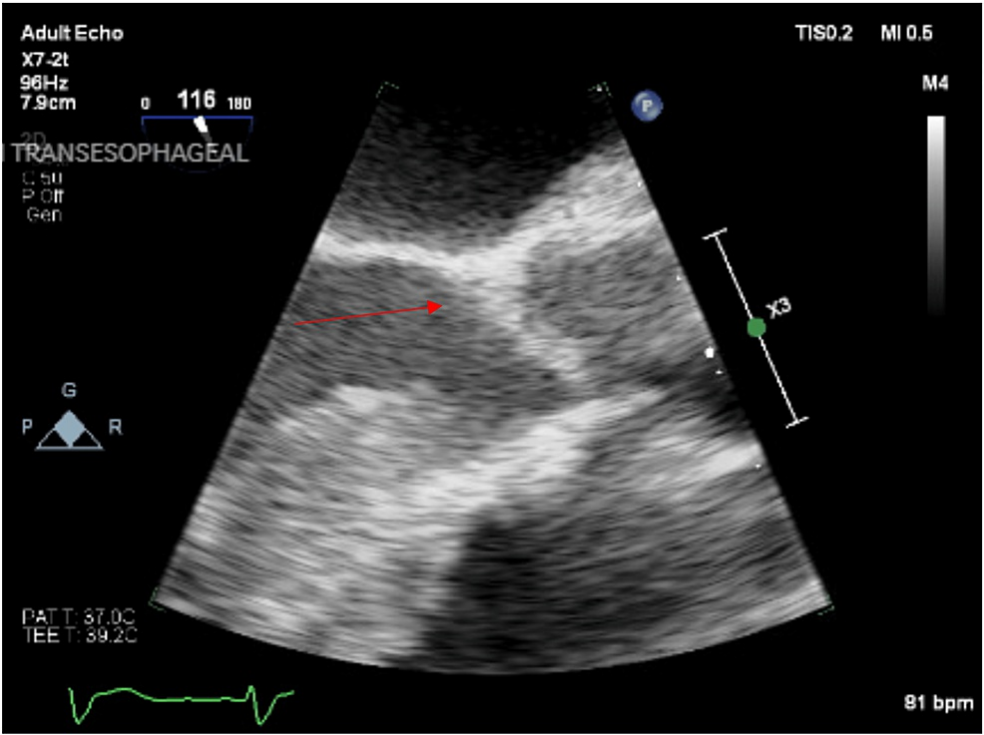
Transesophageal echocardiography. A small, loosely organized, mobile vegetation (red arrow) on the aortic valve
